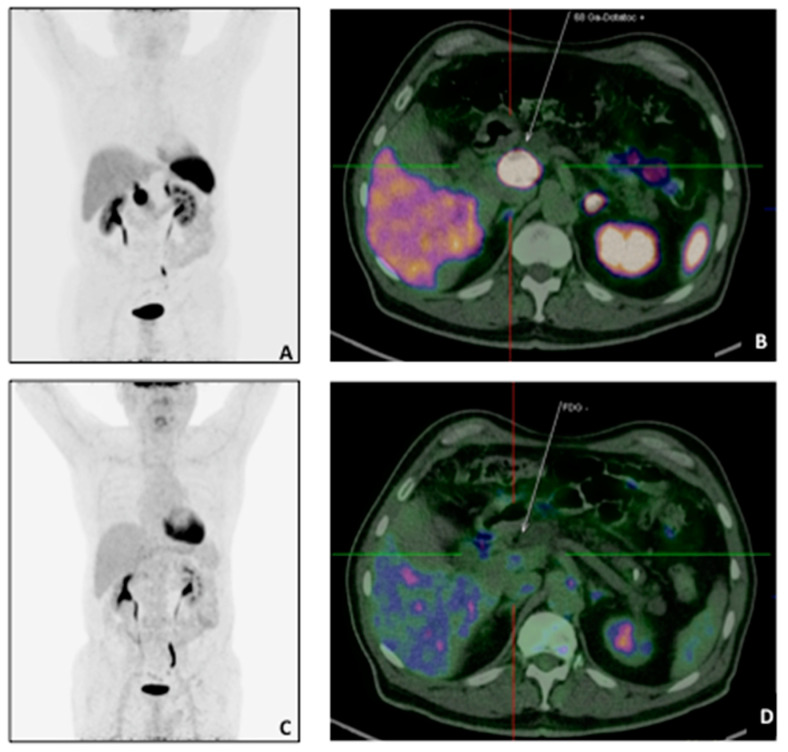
A 73 years-old patient with pancreatic NET (G2, pT2N1M0) underwent 68Ga-DOTATATE and 18F-FDG PET/CT for staging purpose. The68Ga-DOTATATE MIP (A) and transaxial sections (B) showed intense uptake in correspondence of the primary tumour (white arrow: SUVmax: 107.1) with a negative 18F-FDG scan (MIP: (C); transaxial: (D).
